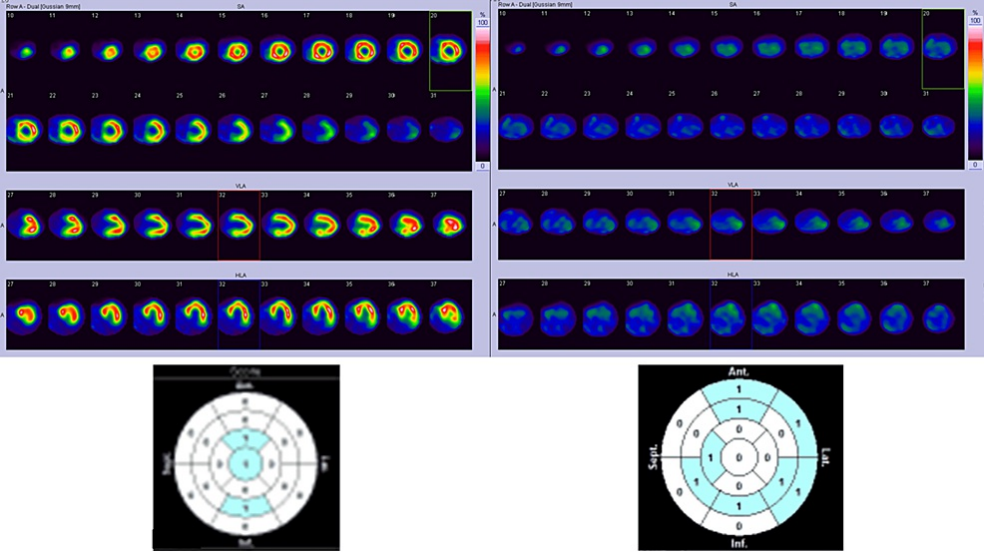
Tl/BMIPP dual-isotope myocardial scintigraphy of Case B. Top left: ^201^Tl myocardial SPECT. Top right: ^123^I-BMIPP myocardial SPECT. Bottom left: Heart Risk View-S scores for ^201^Tl uptake reduction displayed in 17-segments map. Bottom right: Heart Risk View-S scores for ^123^I-BMIPP uptake reduction displayed in 17-segments map. SPECT, single photon emission computed tomography; ^123^I-BMIPP, ^123^I-β-methyl-P-iodophenyl-pentadecanoic acid
